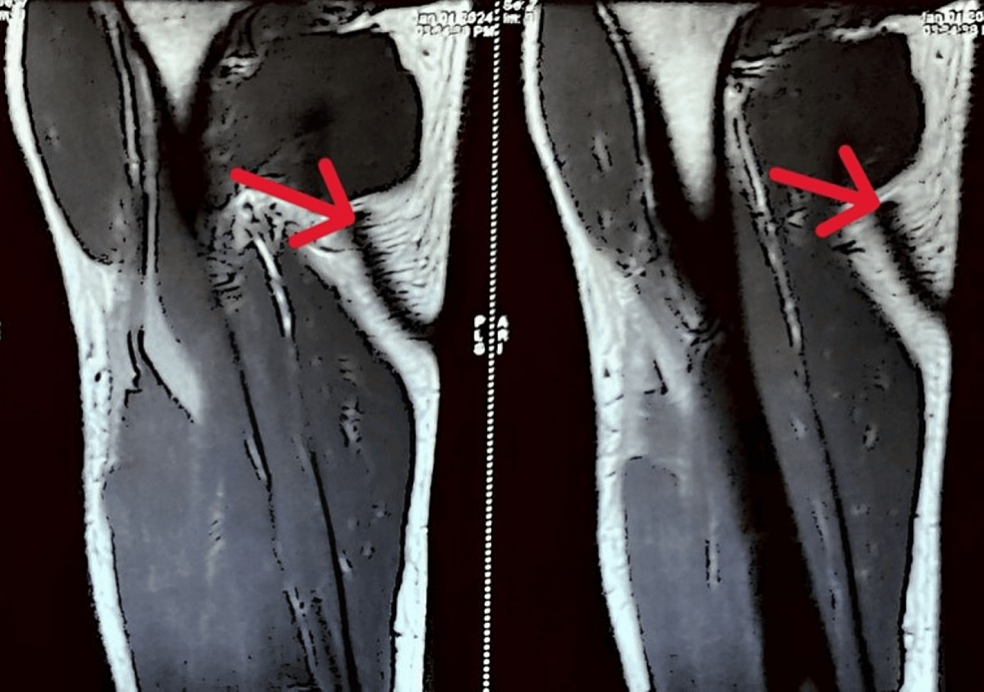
MRI showing the tear of the long head of the biceps tendon The red arrow shows the site of the tear.
